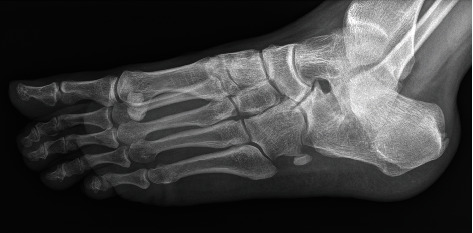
Oblique x-ray image of the left foot shows irregularity of the cubonavicular and calcaneonavicular articular surfaces with sclerotic changes.
